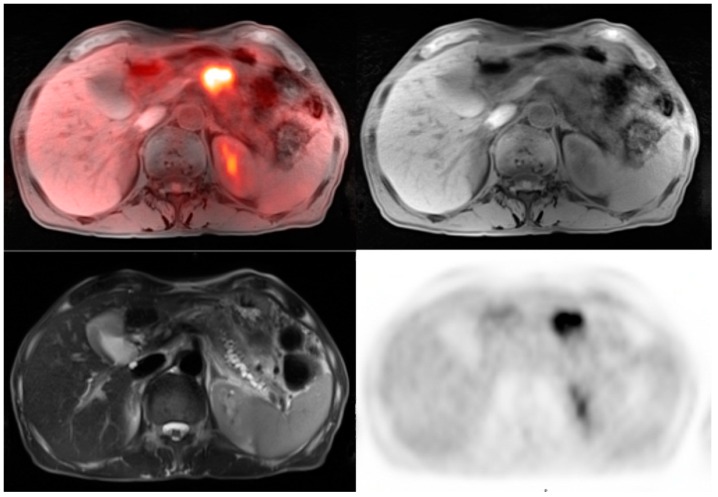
62-year-old male for initial staging of pancreatic adenocarcinoma. The PET-MRI axial fusion image shows a large hypermetabolic mass in the body of the pancreas (*top left*). The T1 axial image demonstrates a mass in the pancreatic body that is isointense to the normal pancreatic tissue (*top right*). The T2 axial images show considerable main pancreatic duct dilatation (*bottom left*). The PET axial image displays intense metabolic activity within the pancreatic tumor relative to the liver activity with standardized uptake value of 8.7.
